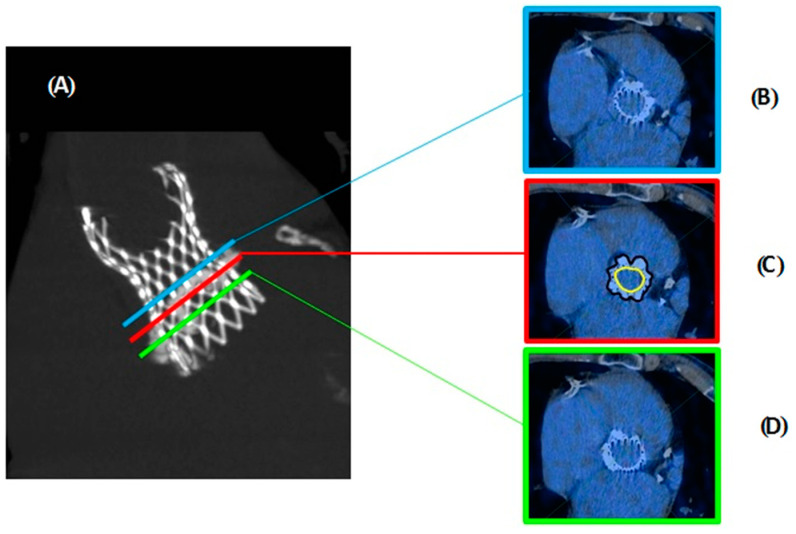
The methodology of leaflet ROI measurement in PET/CT examinations (A)—TAVI Evolut R Valve on CT—image used for estimation of leaflets localization and the choice of ROI levels. The top, middle, bottom cross-section of value used for measurements were marked with colors; Cross-sections of the valve on PET/CT examination (B)—on the top level (blue color); (C)—middle level (red color), yellow and black contour show method of inner and outer outline (ROI) for SUV measurements; (D)—bottom level (green color).
